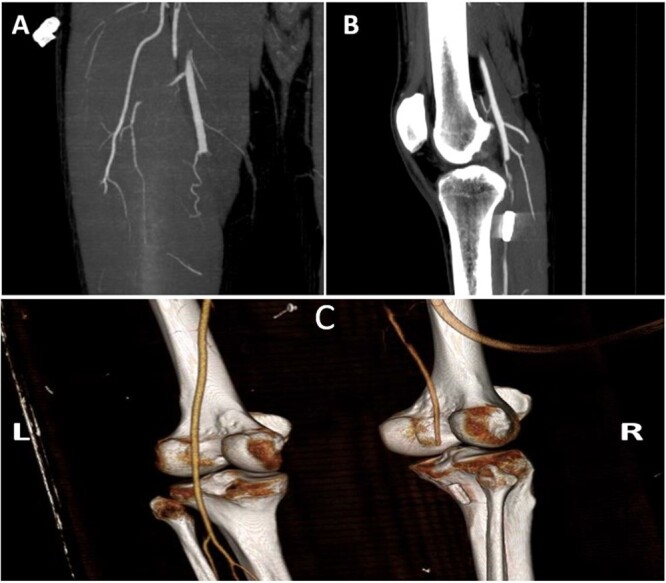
Maximum intensity projection images of computed tomography angiography of the lower limb showing (A) total occlusion of the mid and distal segments of the right superficial femoral artery and (B) showing occlusion of B2 and B3 segments of the popliteal artery with the bullet distally. (C) Volume-rendered computed tomography angiographic images of the lower limbs with a bullet behind the popliteal surface of the right tibia along with occlusion of the popliteal artery proximally.
